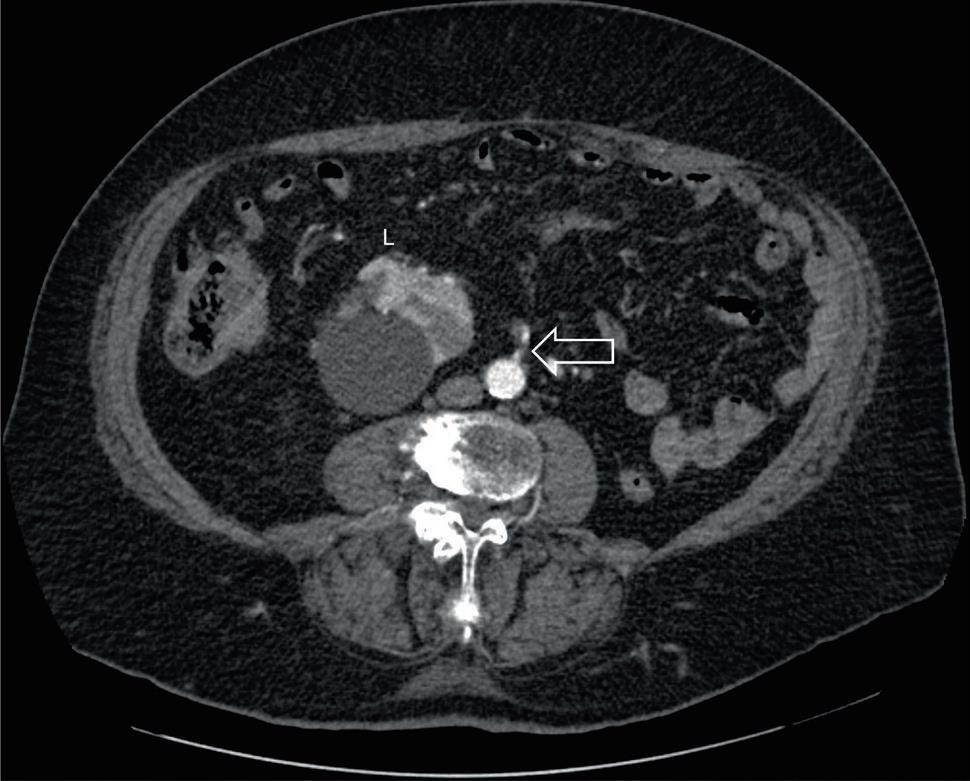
Axial view of arterial phase CT‐urogram. White arrow indicates the left main renal artery originating from the left anterior wall of the abdominal aorta. Left ectopic kidney is marked by white “L”. The left kidney is malrotated with the hilum facing right and posterior, away from the midline. A large, benign renal cyst lies lateral to the left kidney′s hilum.
